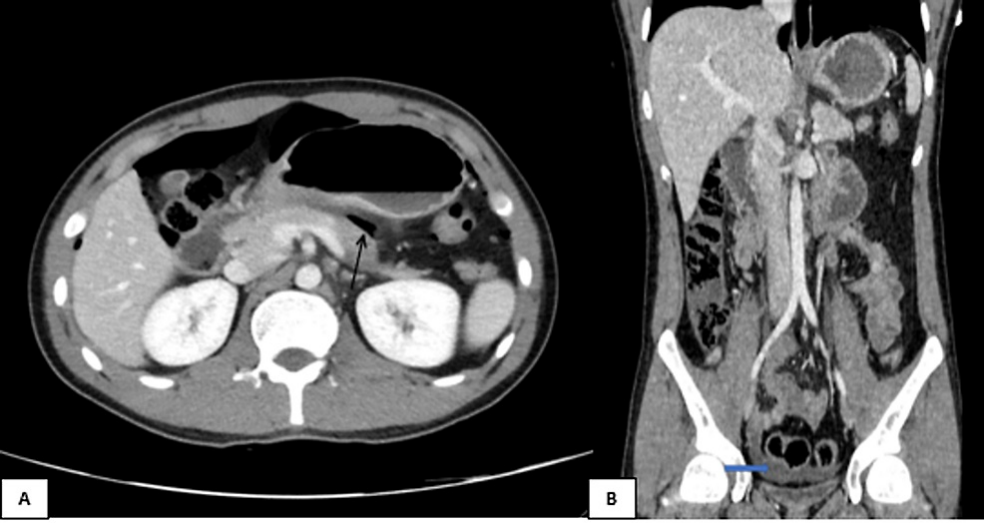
CT abdomen with IV contrast (A) axial and (B) coronal reformatted images showing pneumoperitoneum with free air in the lesser sac (black arrow) and free fluid in the pelvis (blue arrow).
